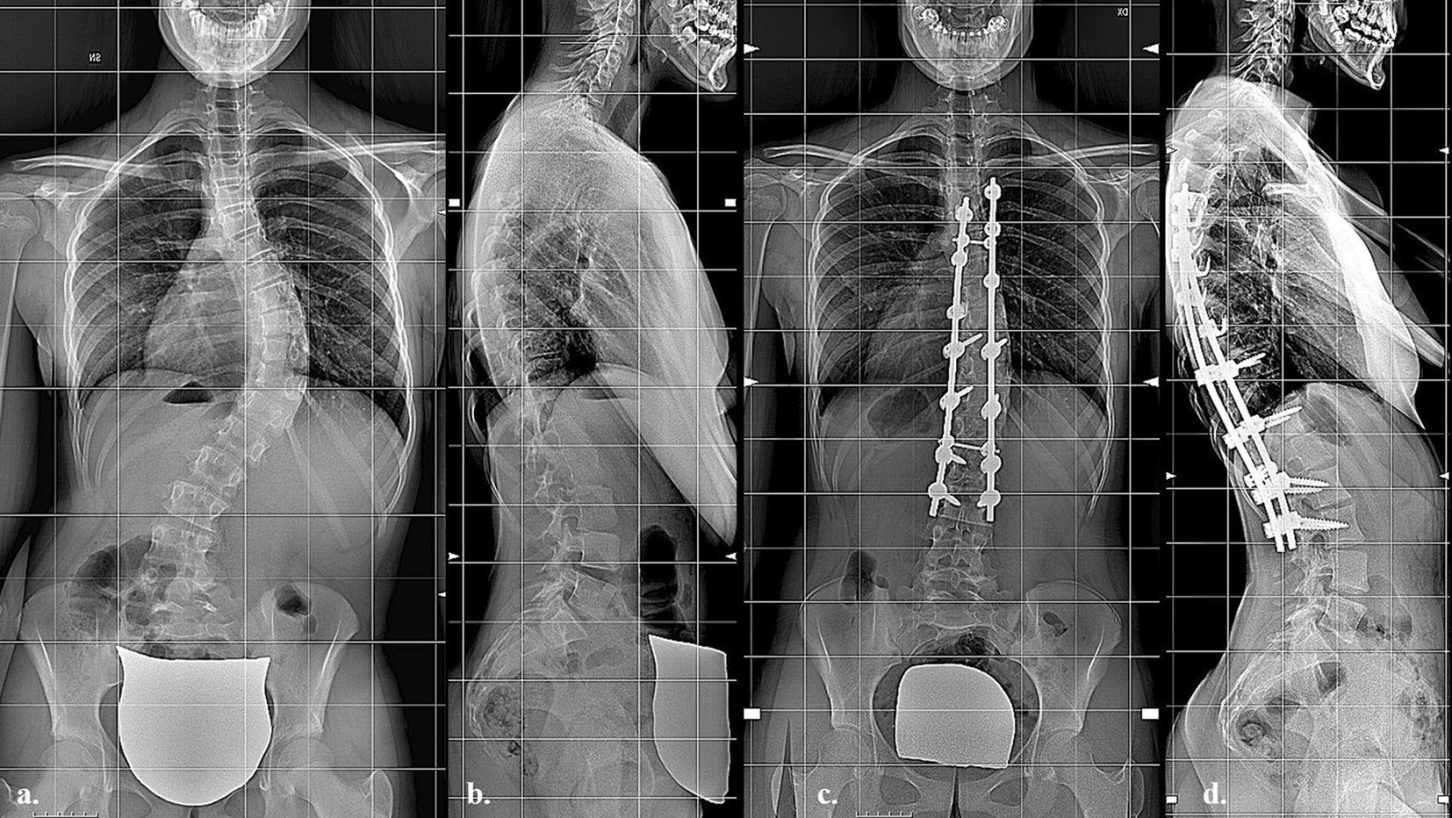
(a) Anteroposterior pre-operative long-cassette X-Ray of a 1C-Adolescent Idiopathic Scoliosis in a 15 years patients with Risser 3; (b) lateral pre-operative long-cassette X-ray showing hypokyphosis < 20°, (c) post-operative anteroposterior long-cassette X-ray showing satisfactory correction with hybrid construct, (d) lateral post-operative long-cassette X-ray showing restoration of normal kyphosis.
